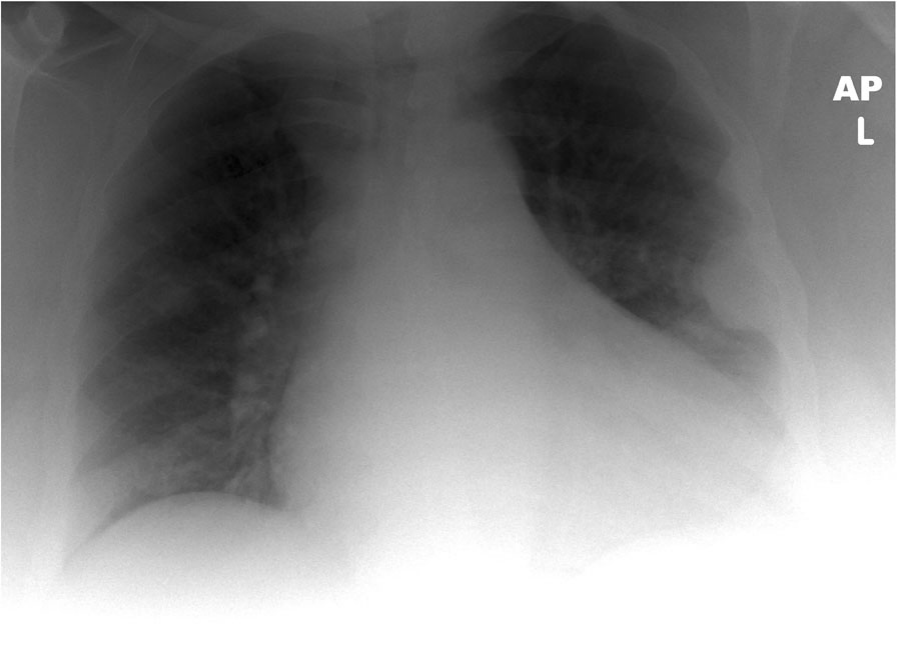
Chest radiograph of the same patient showed sclerotic expansion of the left lateral ribs
